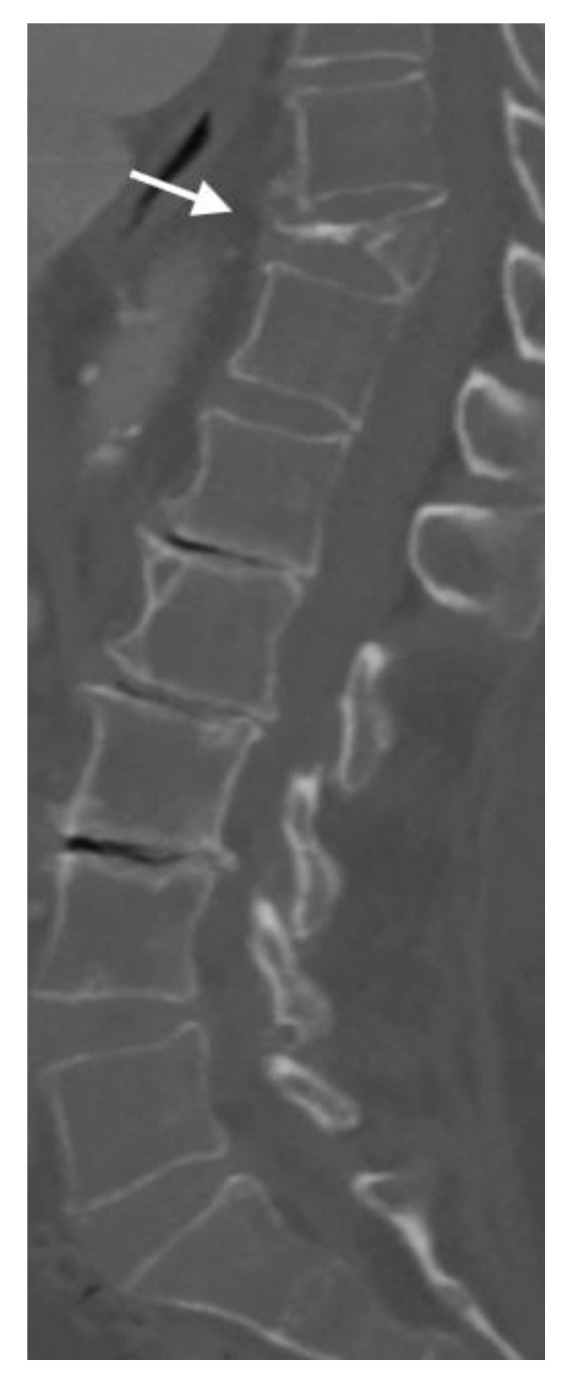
In this sagittal CT, the arrow shows severe T11 vertebral compression fracture, Grade 3 according to Genant classification. The bones are diffusely demineralized.
